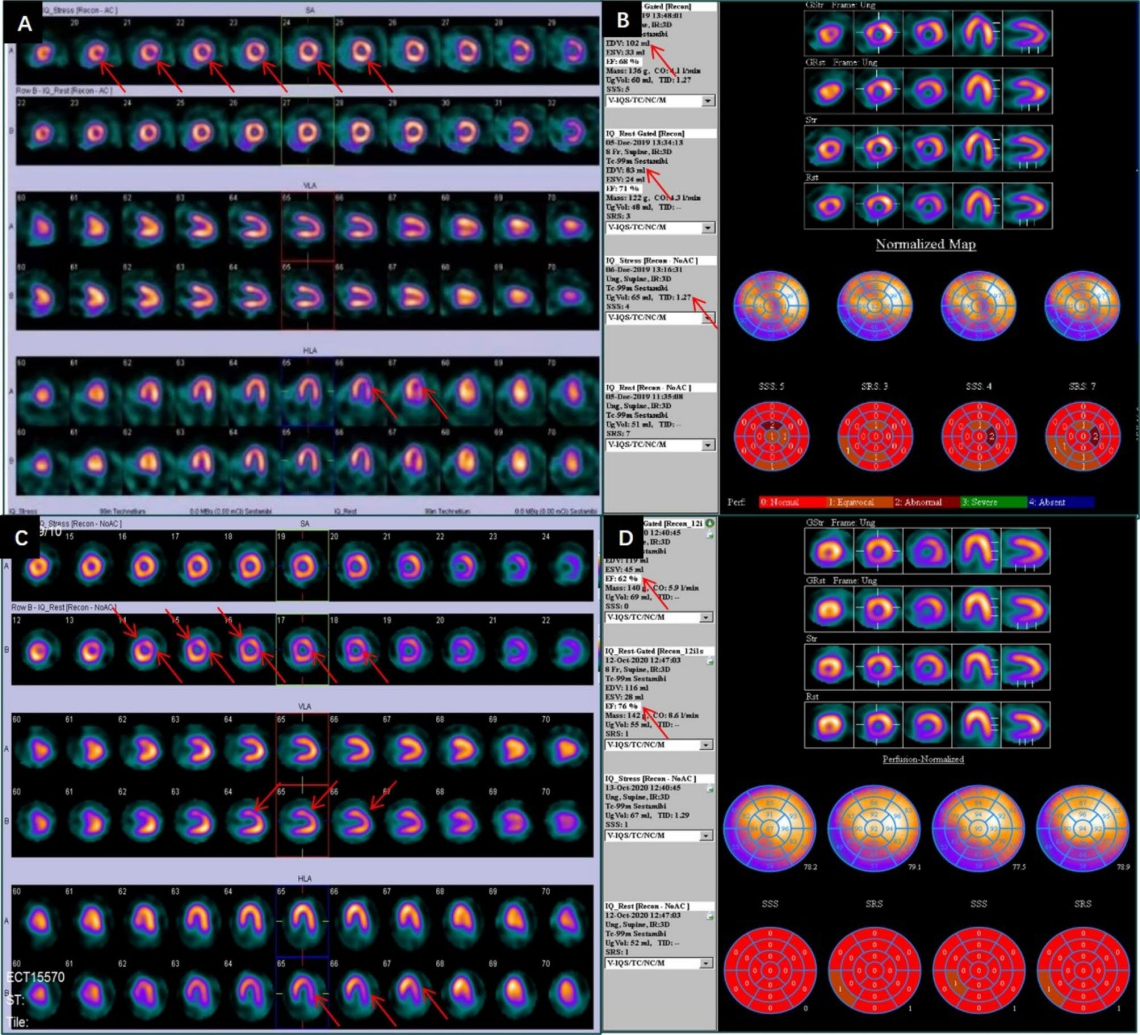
Four abnormal SPECT phenomena (A) RMPD: The stress SPECT images show a reversible perfusion defect from the lateral wall apex to part of the middle of the posterior lateral wall. (B) TID: Rest EDV is 83ml and stress EDV is 102ml. The TID value is 1.27, which is likely to represent diffuse subendocardial ischemia or microvascular disease in the absence of epicardial coronary disease. (C) RR: The stress images show no perfusion defects, while the rest images show perfusion defects (including in the apical anterior segment of the left ventricle wall, the lateral apical segment, the posterior lateral wall mid-segment, and the basal segment). (D) EF decline ≥ 5%: Rest EF was 76%, and stress EF is declined to 62%; EF declined 14%
